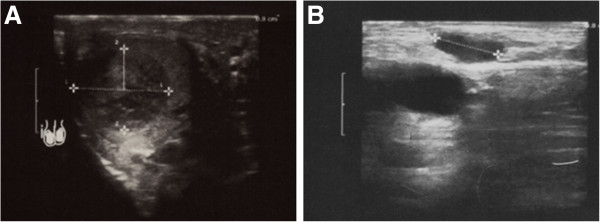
Ultrasound images show a solid and inhomogeneous hypoechoic mass in the upper lobe of the right testis (A) and a right inguinal lymphoadenopathy 2 cm in diameter (B).
